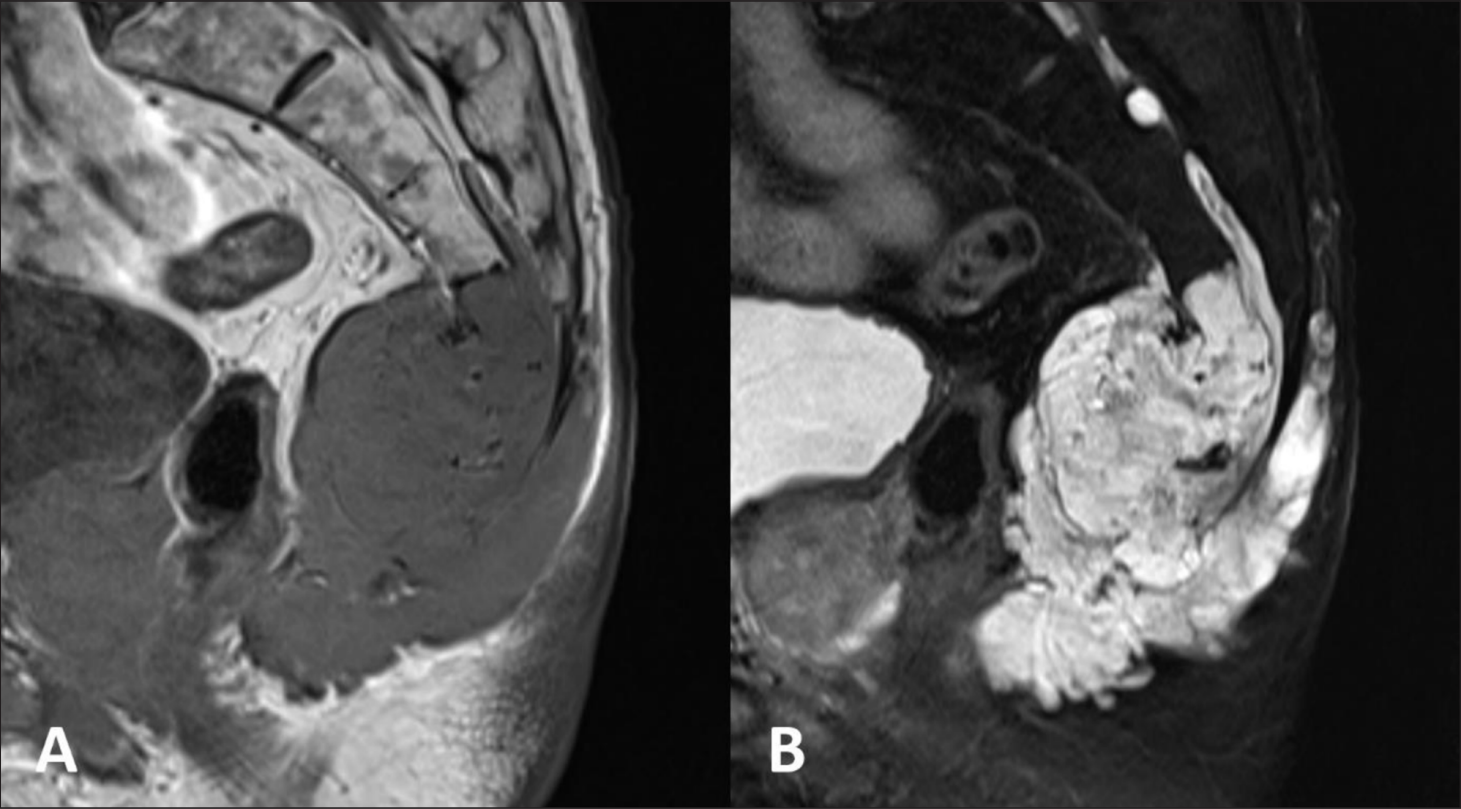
A 79-year-old male with sacral chordoma. (a) Sagittal T1-weighted MR image shows a hypointense mass arising from the lower sacrum with a large soft tissue component. (b) Sagittal T2-weighted MR image shows a lobulated mass with a heterogeneous hyperintense signal, invading the bone and soft tissues.
